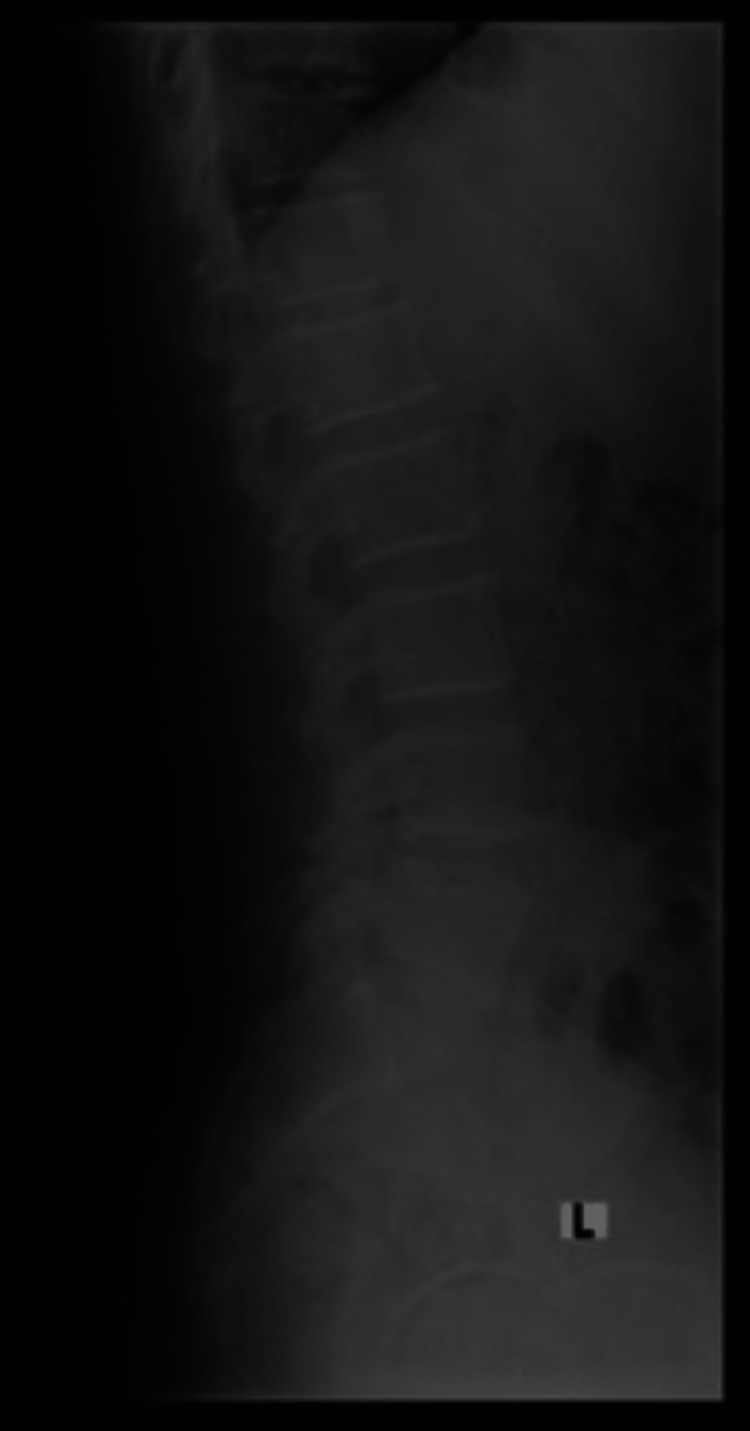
Thoracic radiograph showing loss of spinal curvature.
